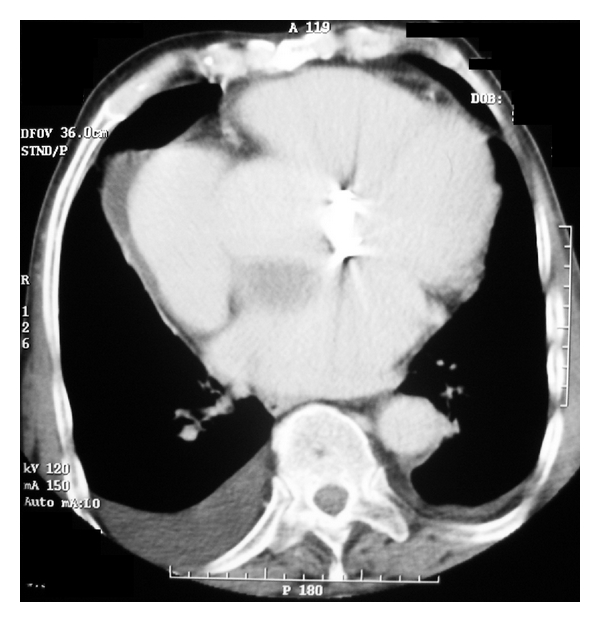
CT scan of the chest revealed pleural effusion at right and left hemithorax and prosthetic aortic valve.
